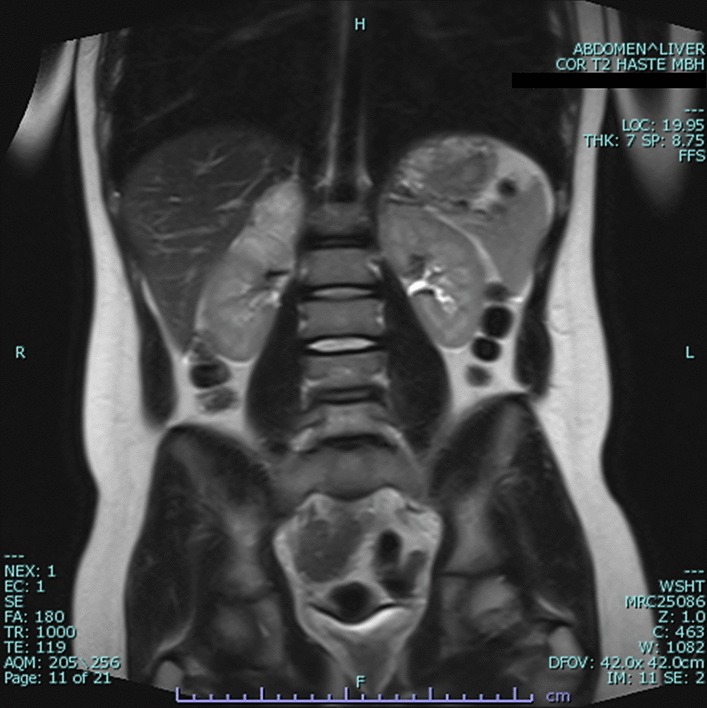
MRI for *Case 2* showing a 6.0 cm × 3.6 cm × 3.2 cm solid enhancing structure in the right adrenal gland region suggestive of a pheochromocytoma
